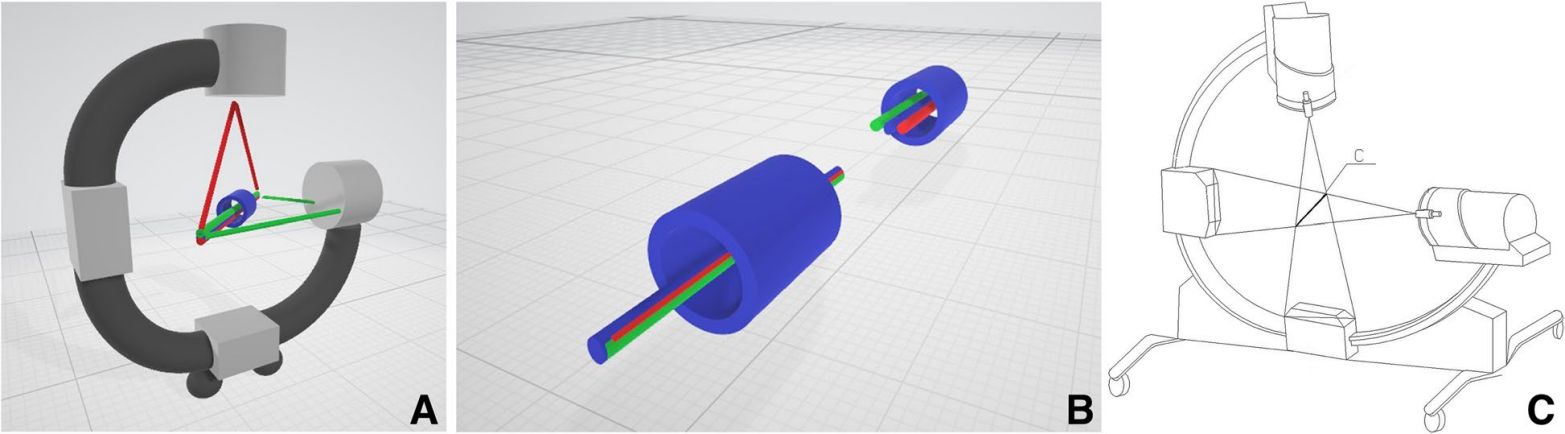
Work principle sketch maps of the laser guiding navigation device (A). This meant that the horizontal plane X-ray central fluoroscopy axis of G arm (the green line), vertical plane X-ray central fluoroscopy axis (the red line) and the target screw passage central axis (the blue line) remain coaxial, known as “three-line coaxial” (B, C). The blue tube represented target channel (B)
